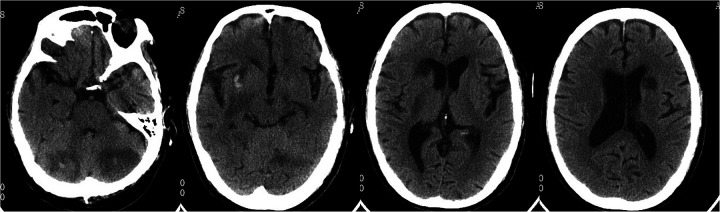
Follow-up head CT revealed multiple cerebral infarctions in the bilateral periventricular regions, basal ganglia, and cerebellum, with a small amount of bleeding in the right basal ganglia infarction area.
